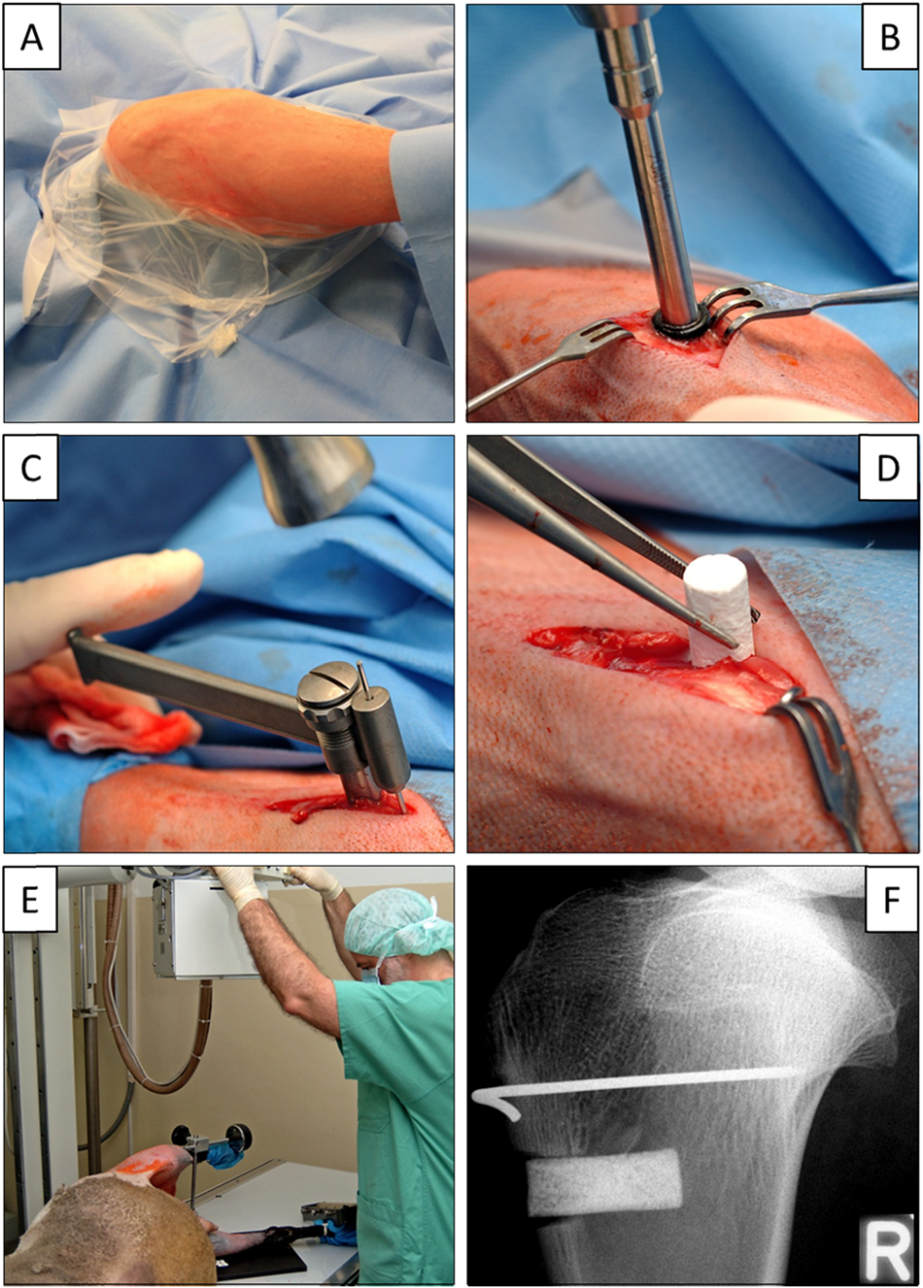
Surgical technique. A Stifle joint operation situs following disinfection and sterile covering of upper and lower limb by a surgical drape; B Skin incision of 40 mm; identification of the appropriate defect position on the medial surface of the right tibial head at the horizontal level of the tibial tuberosity (half distance between tuberosity and posterior edge of the tibia; center of the visible medial surface); defect generation with an orthograde orientation using a drill (8 mm trephine; Stryker); C, D Defect placement of the implant using Kirschner guide wire and surgical adapter; E, F X-ray imaging to exclude either implant protrusion from the tibial surface or sinking into the depth of the tibia
